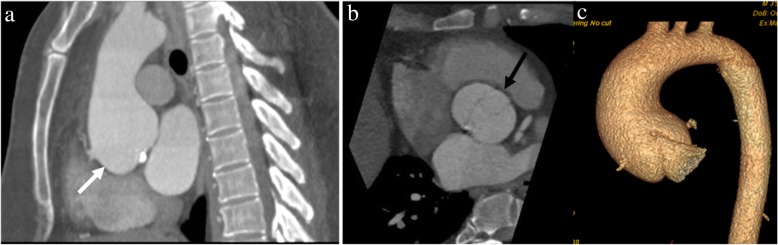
A 43-year-old man with Marfan syndrome and bicuspid aortic valve: sagittal (a) and axial (b) CTA images shows aortic root aneurysm with dilatation centered at the sinuses of Valsalva (white arrow in a) with effacement of the sinotubular junction and normal caliber ascending aorta and bicuspid aortic valve (black arrow in b). A “tulip-shaped” configuration of the aortic root is better appreciated on the volume rendered image (c)
